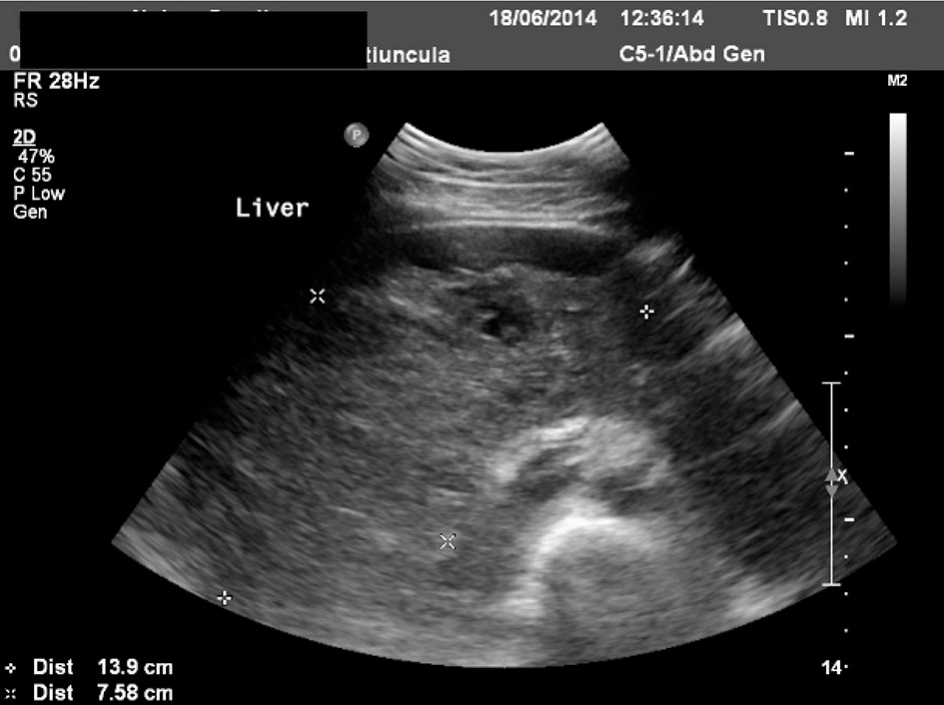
Ultrasonography demonstrated an echogenic collection in gallbladder fossa measuring 13 × 7 cm.
